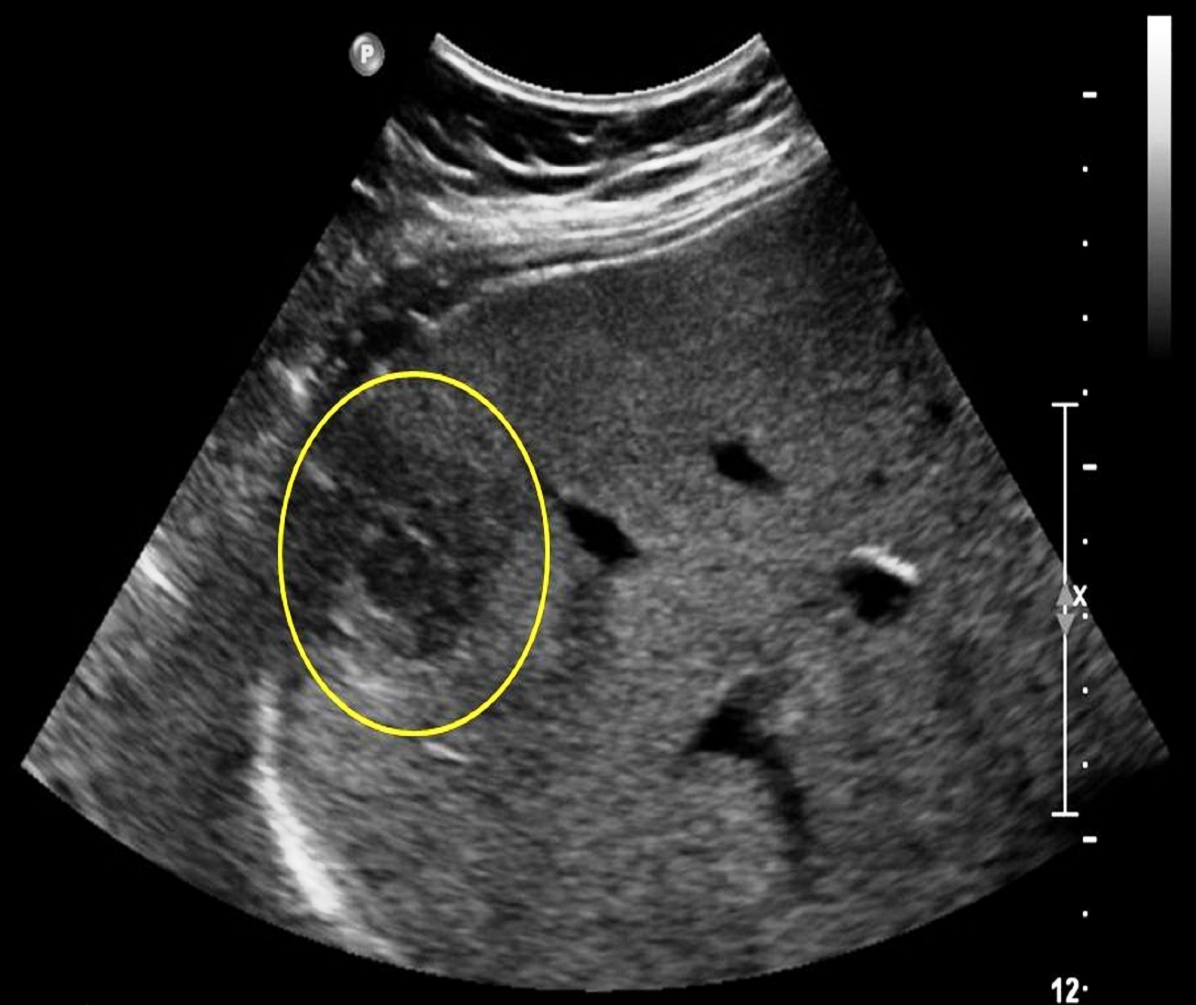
Ultrasonography RUQ A 15.4-cm lesion in the RMCL with a smooth contour and mildly heterogeneous echogenicity was identified. A 4.7 x 2.7 x 2.6 cm mostly hypoechoic, ovoid mass in the inferior aspect of the posterior right hepatic lobe contained a 1.6 x 1.1 x 1.3 cm focal hypoechoic structure with a mildly hyperechoic rim internally.
